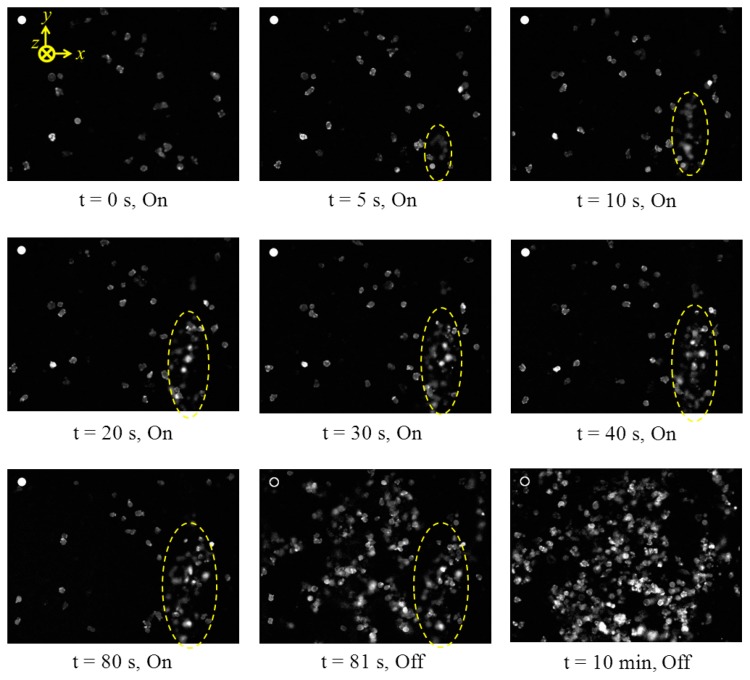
Image sequences of the formation of an agglomeration of cells in the curved ultrasonic resonator field. The circle at the top-left of each image is 10 μm diameter. The filled circle denotes that the transducer is activated.
